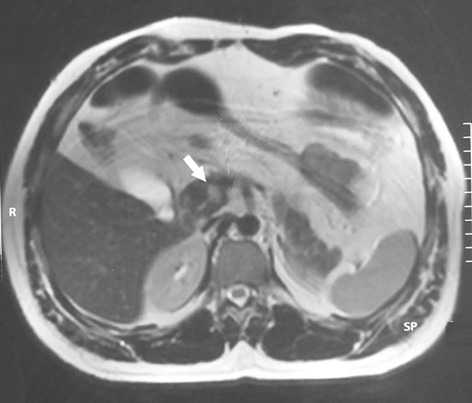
T2-weighted MRI of the abdomen showed a hypervascular lesion of 10mm localized in the head of the pancreas
